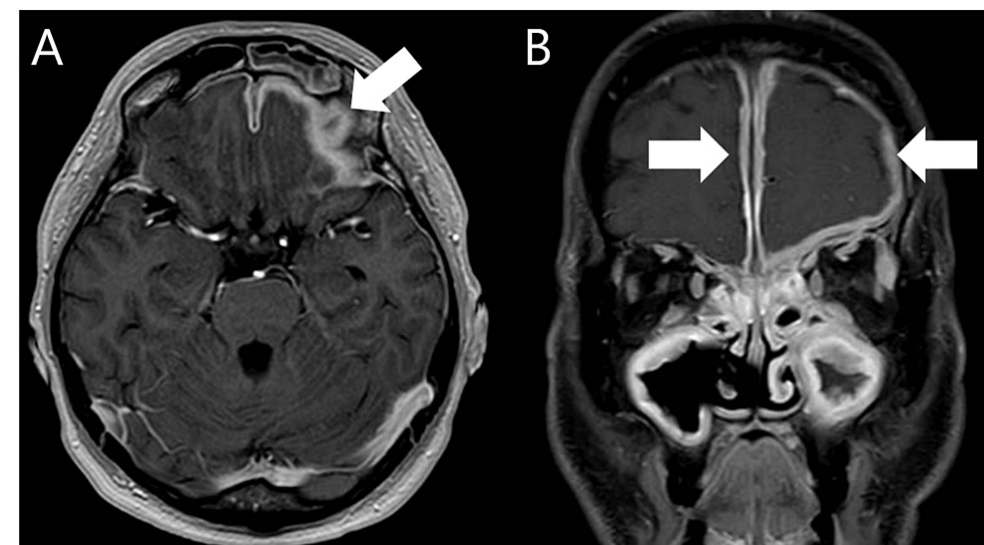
T1-weighted MRI images (left: axial, right coronal). T1-weighted MRI images showed that the dura thickened and contrasted with gadolinium (Gd) (white arrow).
